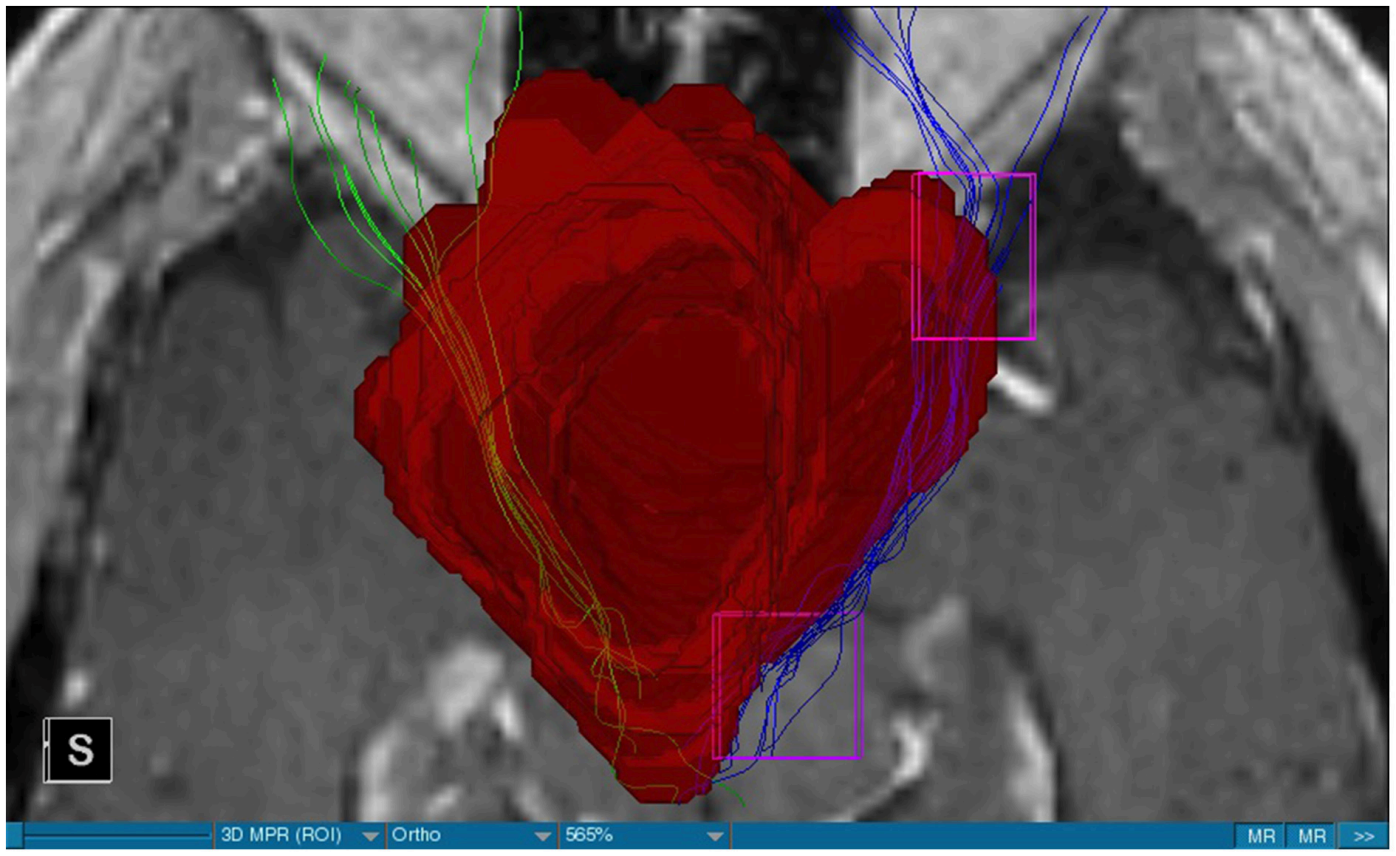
MRI axial view, T1, red: Glioblastoma, blue: right MFB, green: left MFB.
